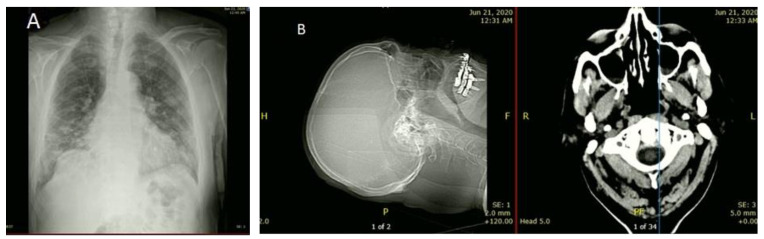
(A) Chest X-ray depicting bilateral multifocal patchy opacity more at lower zone and no cardiomegaly. (B) Brain CT scans showed no acute major infarction or hemorrhage.
